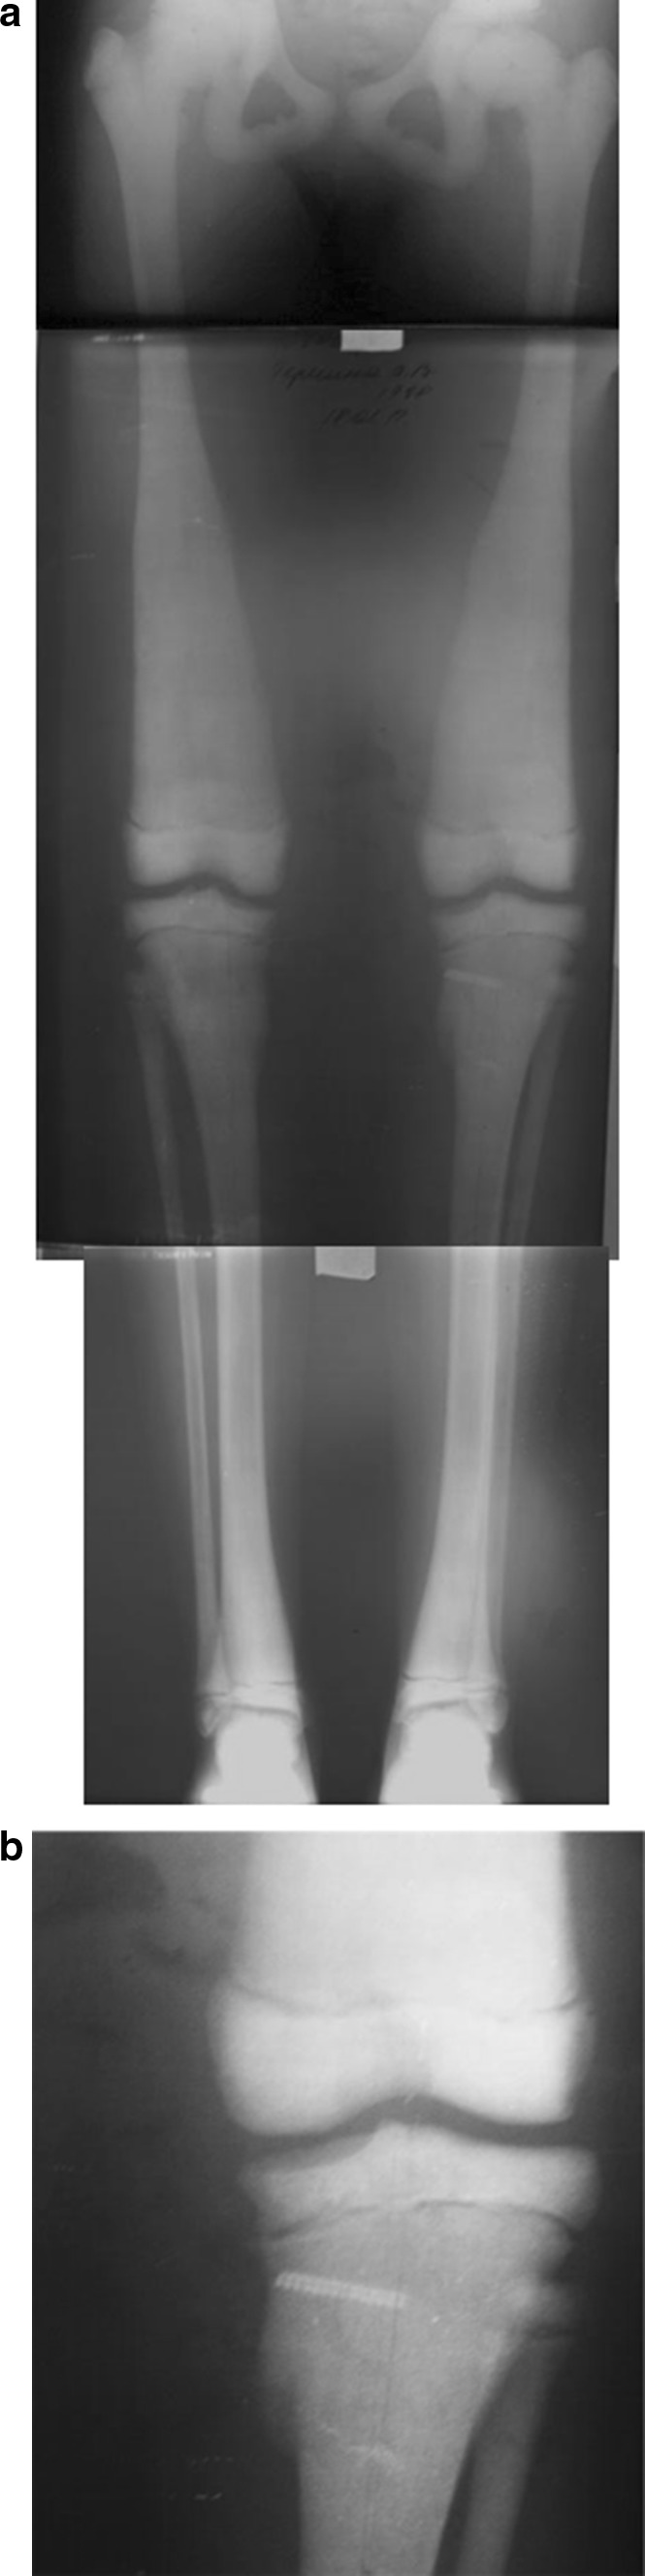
Radiographs after frames removal: a aMPTA 86° (*right side*) and 85° (*left side*); *MAD* medial position of 3 mm to center of right knee joint and 4 mm medial to center of left knee joint; b broken screw in tibial metaphysis
